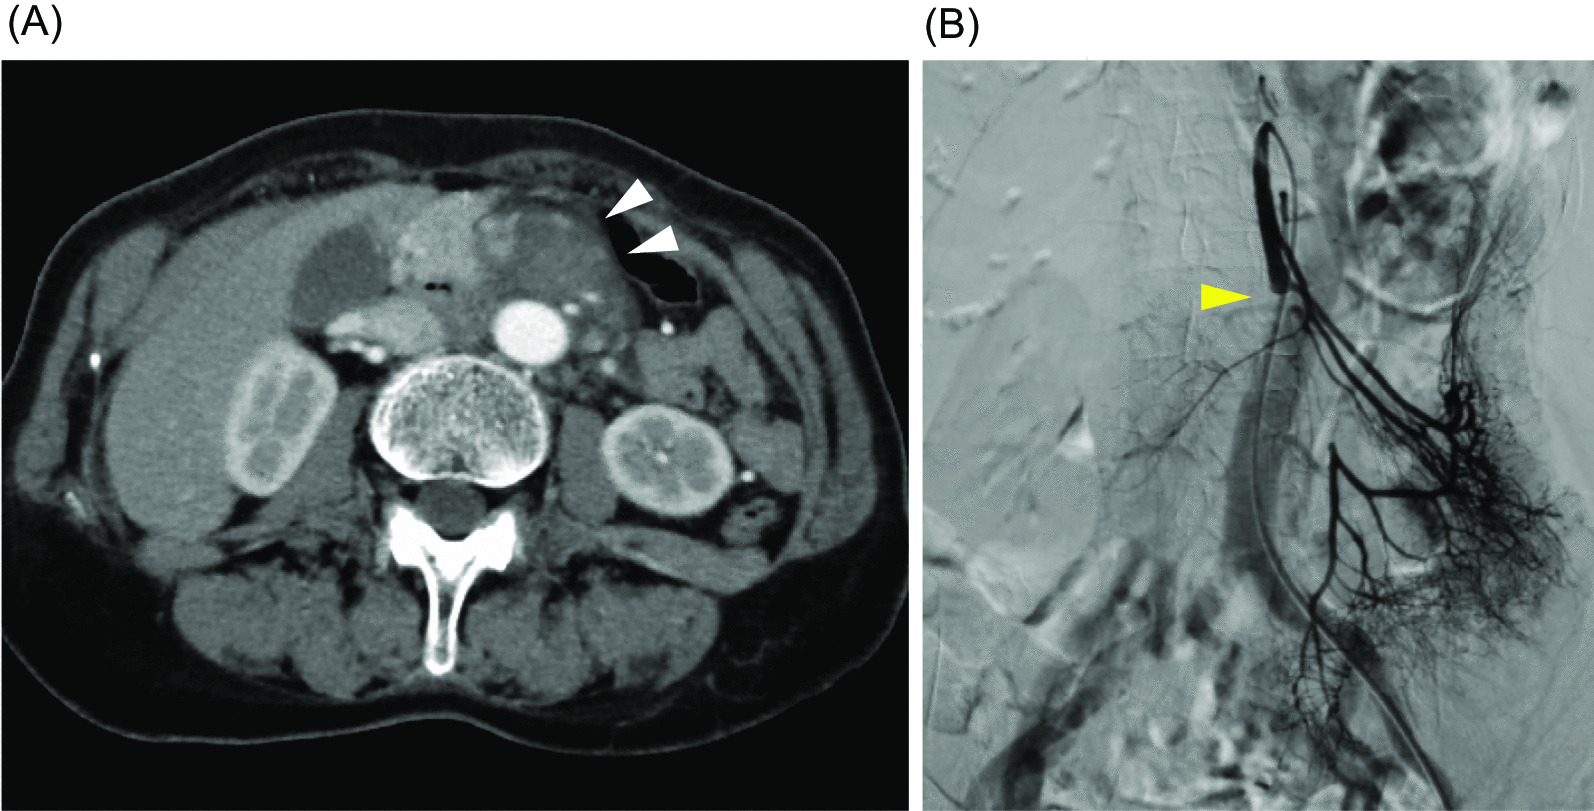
Imaging studies performed at first admission. A Completely obstructed SMA and swollen mesentery (white arrows) in a CT scan. B Abdominal angiography presented total occlusion at the first branch of the SMA (yellow arrow)
